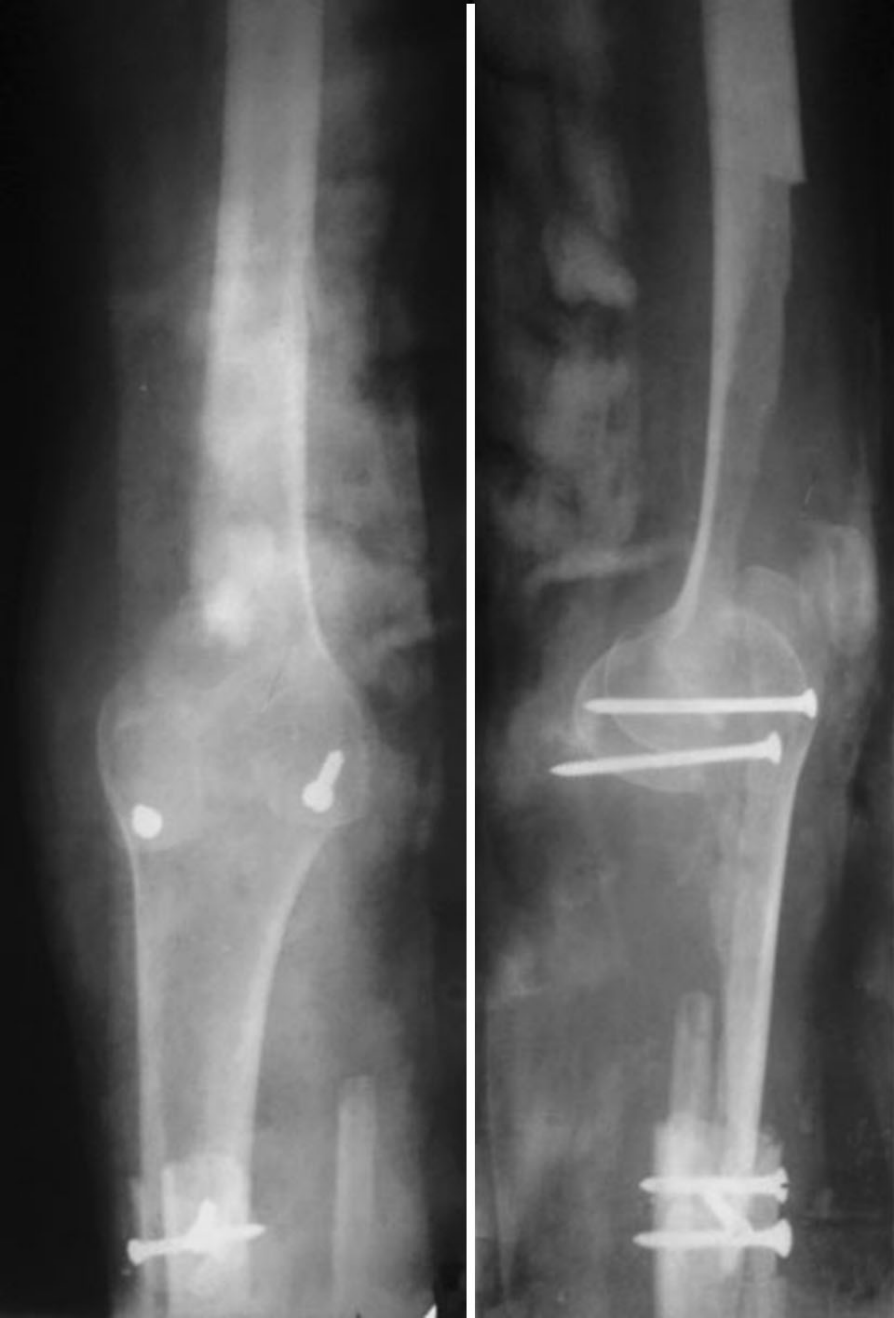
Antero-posterior and lateral radiographs of a 63-year-old male patient (case 13) showing a distal femur turnoplasty being performed for a recurrent lesion in the proximal tibia
